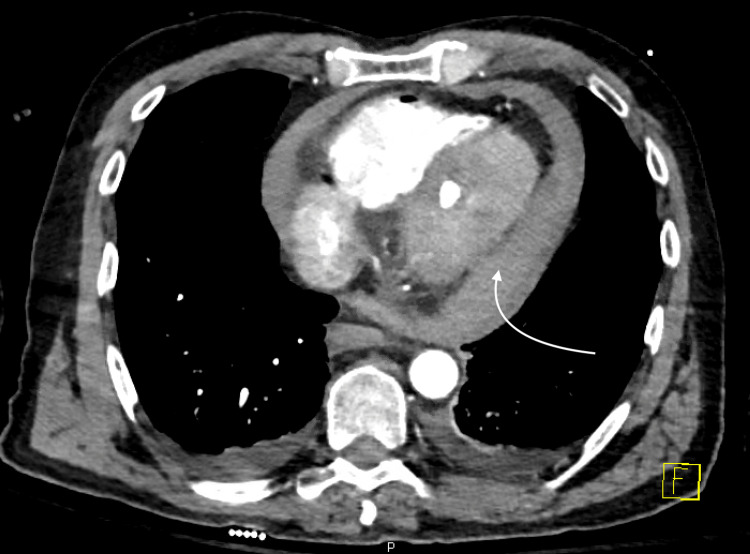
Computed tomography angiography (CTA) chest demonstrating a moderate-size pericardial effusion highlighted by the arrow.
